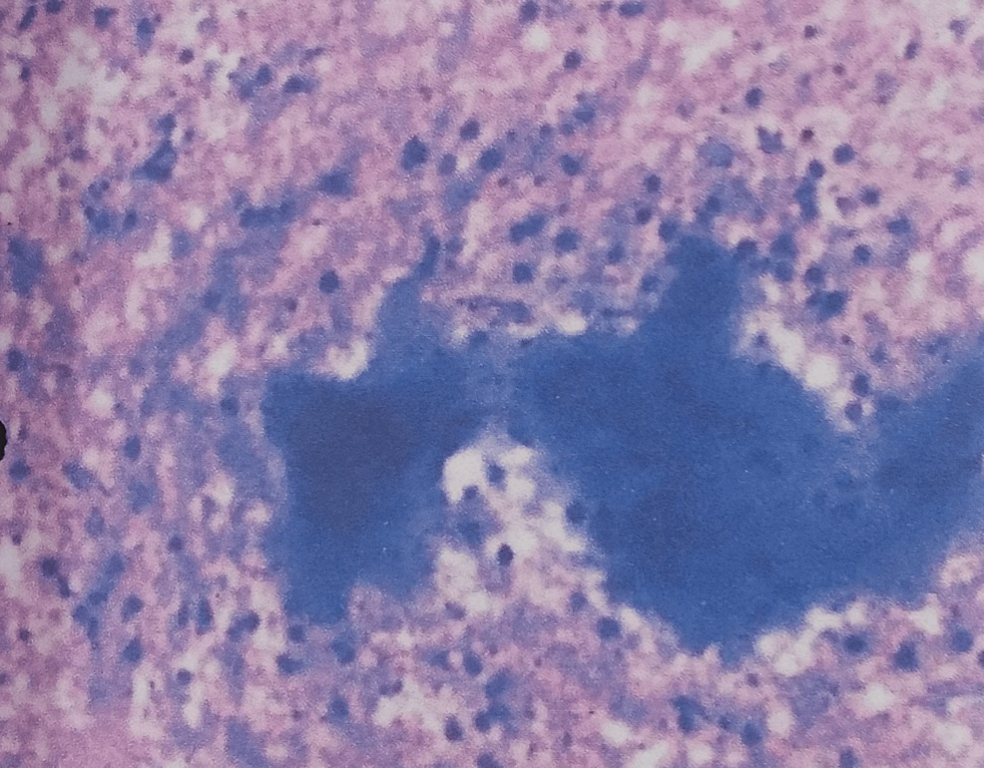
USG-guided FNAC of the pus showing necrosis USG: ultrasound; FNAC: fine-needle aspiration cytology
